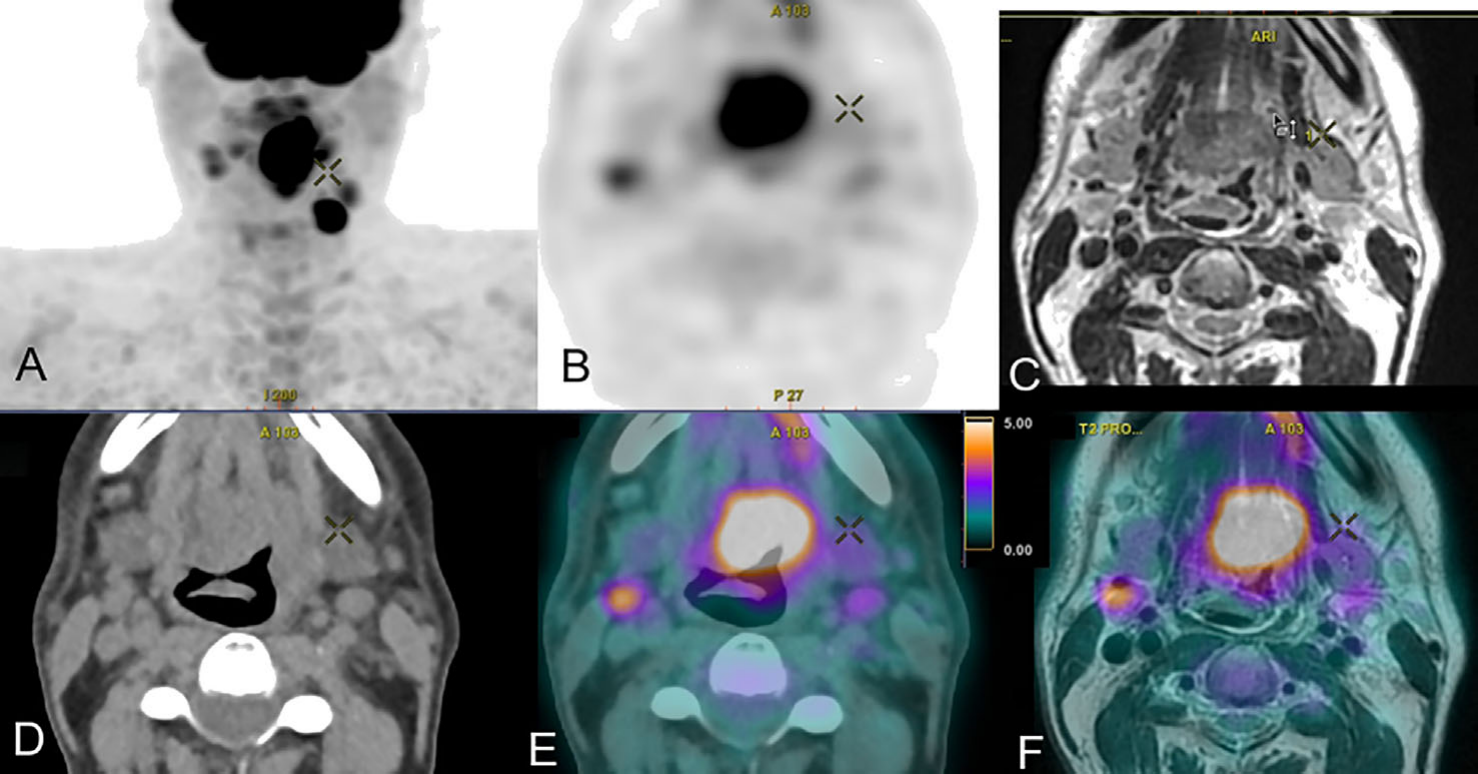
Trimodal acquisition of a cancer of the base of the tongue with in (A) the frontal maximum intensity projection PET image, in (B) the axial PET FDG acquisition, in (C) the axial T2 MRI acquisition, in (D) the axial CT acquisition, in (E) the axial PET/CT fusion and in (F) the axial PET/MRI fusion.
